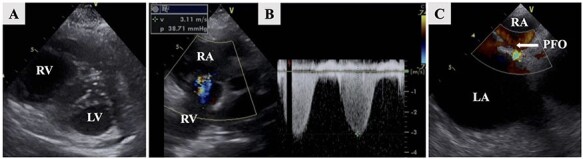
Transthoracic echocardiography shows left ventricular retraction due to right ventricular dilation (A). TR flow was 3.1 m/sec and the TR-PG was 38.7 mmHg (B). Transesophageal echocardiography revealed a PFO and shunt blood flow from the right-to-left atrium (C, arrow).
